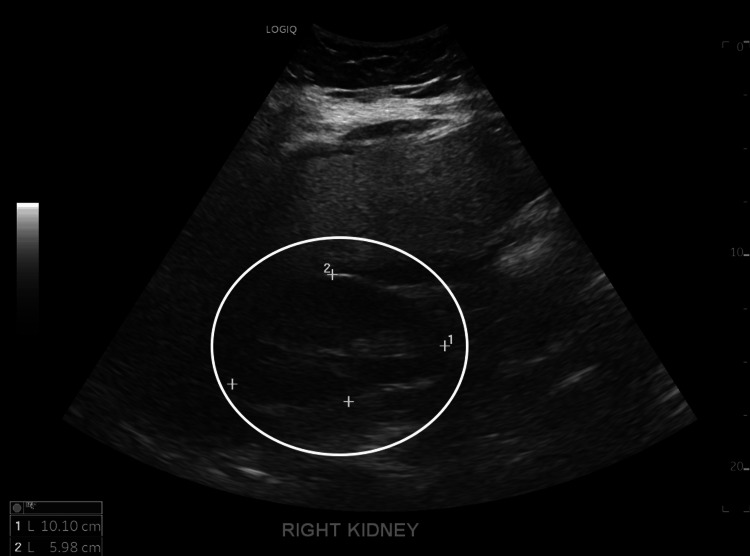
Right kidney ultrasound demonstrates increased echogenicity reflective of active glomerular injury and chronic fibrotic remodeling characteristic of antineutrophil cytoplasmic antibody (ANCA)-associated vasculitis.
